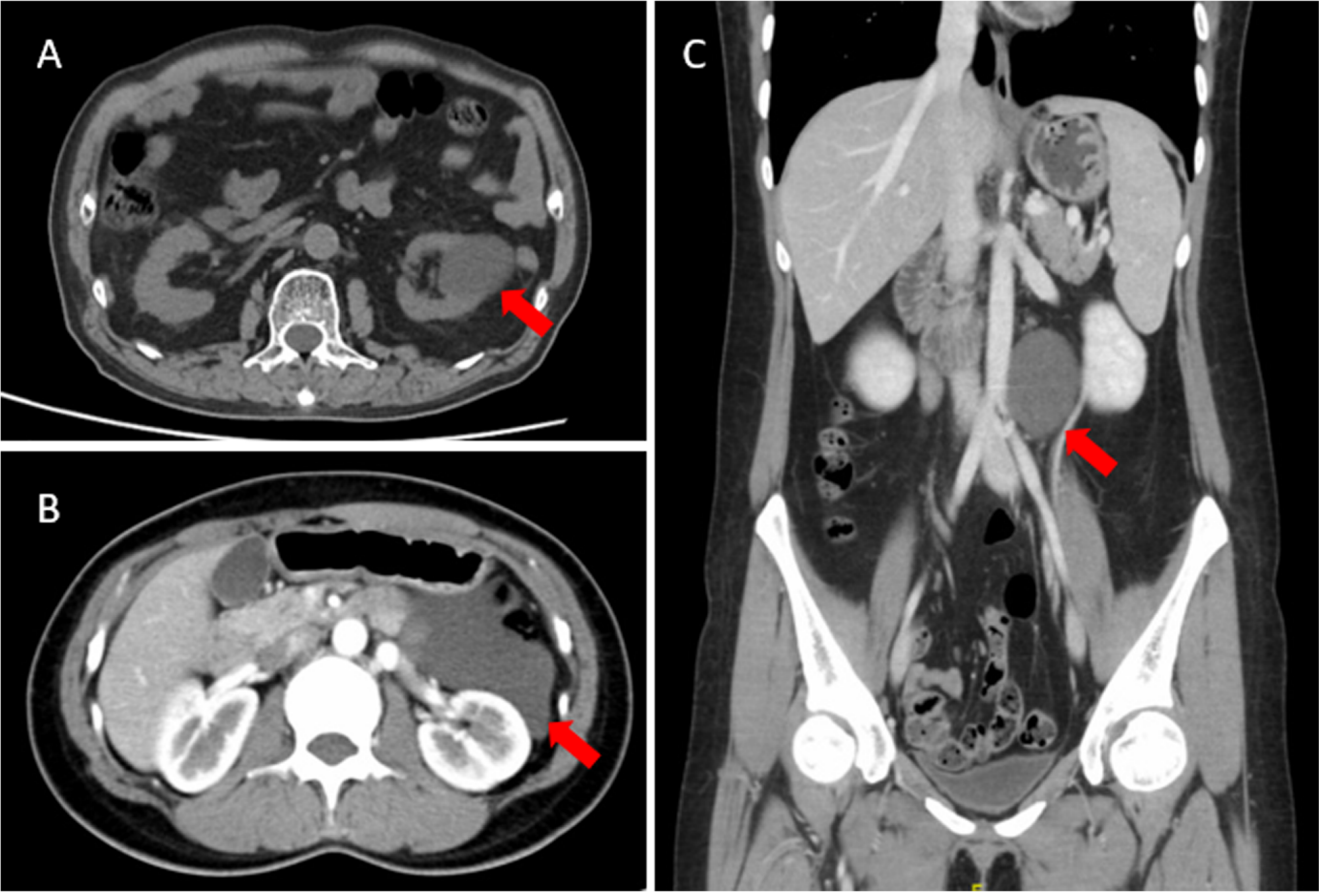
CT scans of Chinese adult patients with ACL. A. ACL close to left renal pelvis of case no. 11 (red arrow).B. ACL between spleen and stomach of case no. 3 (red arrow). C. ACL between aorta and left kidney in three-dimensional CT reconstruction of case no. 12 (red arrow). ACL, abdominal cystic lymphangioma; CT, computed tomography
